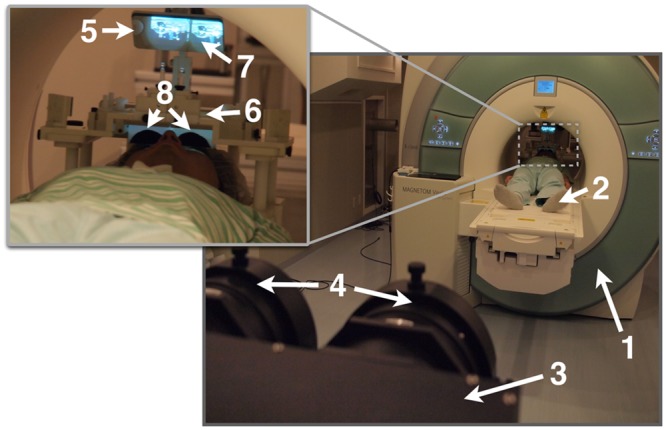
Photograph of apparatus setup for wide-view stereoscopic visual stimulation inside a MRI scanner. (1) MRI scanner, (2) subject, (3) video projectors in electromagnetically shielded box, (4) telescopic lenses for long-distance video projection, (5) front surface mirror, (6) projection screen, (7) stereoscopic visual image on the projection screen viewed via the mirror, and (8) wide-view binocular eyepiece lenses.
